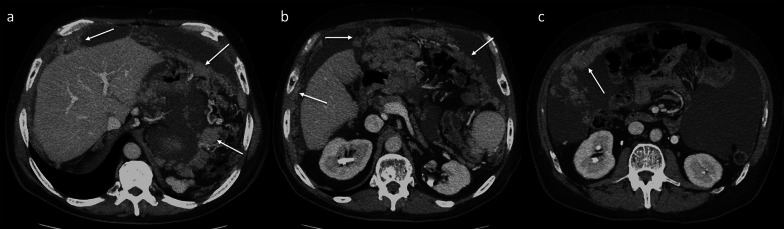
Omental recurrence. Axial contrast-enhanced CT images on the portal venous phase (a–c) show multiple confluent masses located in the peritoneal cavity and omentum (arrows). These lesions coalesce to form a diffuse soft tissue density mass, the so-called omental cake
